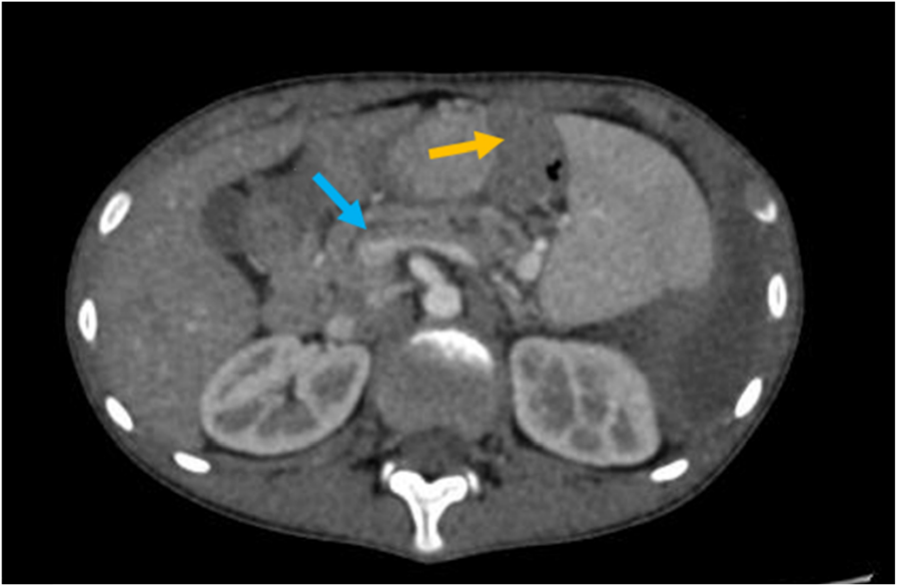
Abdominal CT showed a small low-density lesion at the distal pancreas, accompanied by a dilatation of the main pancreatic duct (blue arrow) and the pancreatic pseudocyst (yellow arrow)
